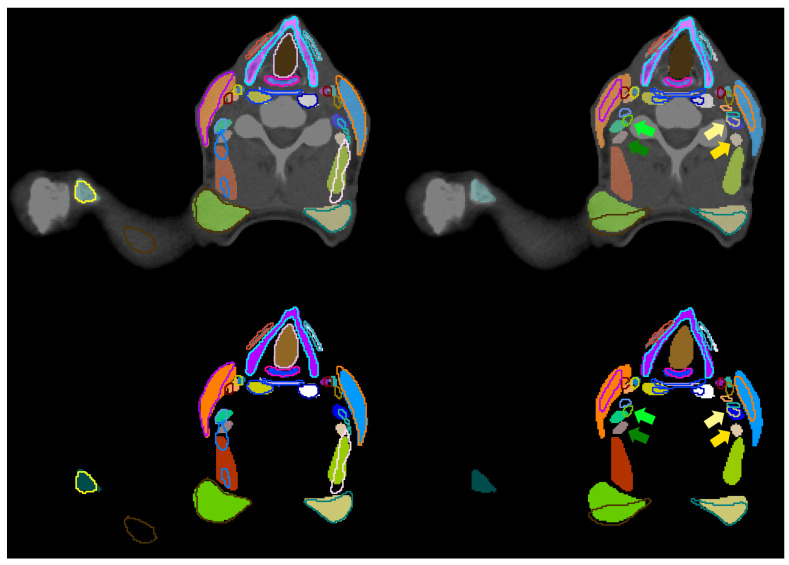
CT slice (top) with contours generated manually (area) for comparison (outline) with contours predicted by our trained nnU-Net models (left), and contours manually delineated by another trained observer (right). The second set of contours does not contain all 71 structures (no outlines). Green (right) and yellow (left) arrows point to corresponding segmentations of the posterior scalene muscle generated by one observer (darker color) or the other (lighter color). The same contours whiteout CT slice are visualized in the bottom row.
